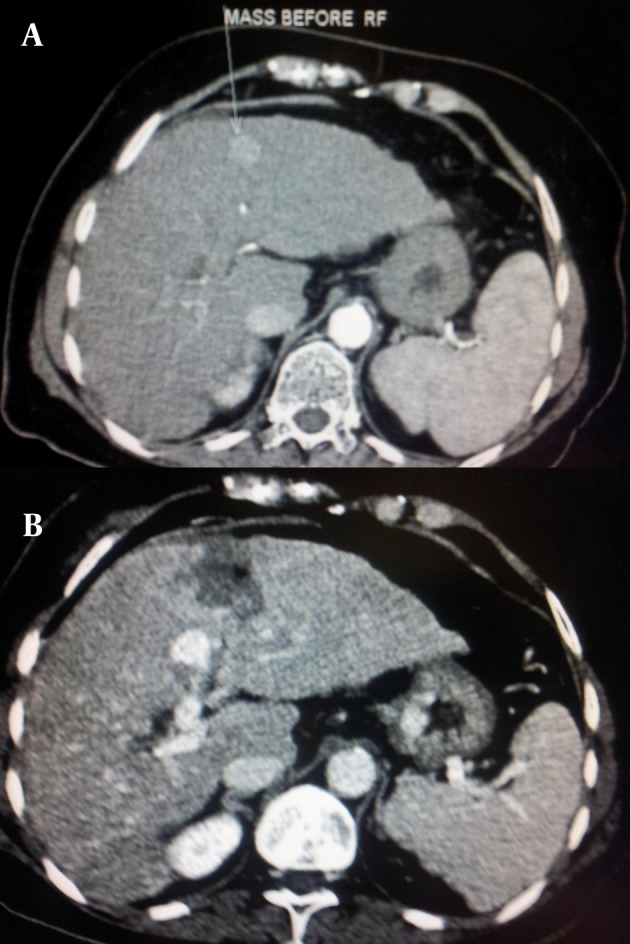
A, Dynamic CT scan, arterial phase shows hyperdense high vascular mass in the 4th segment of the liver; B, Dynamic CT scan, arterial phase, 5 min after RFA, shows a hypovascular area and complete vanishing of the enhancement.
